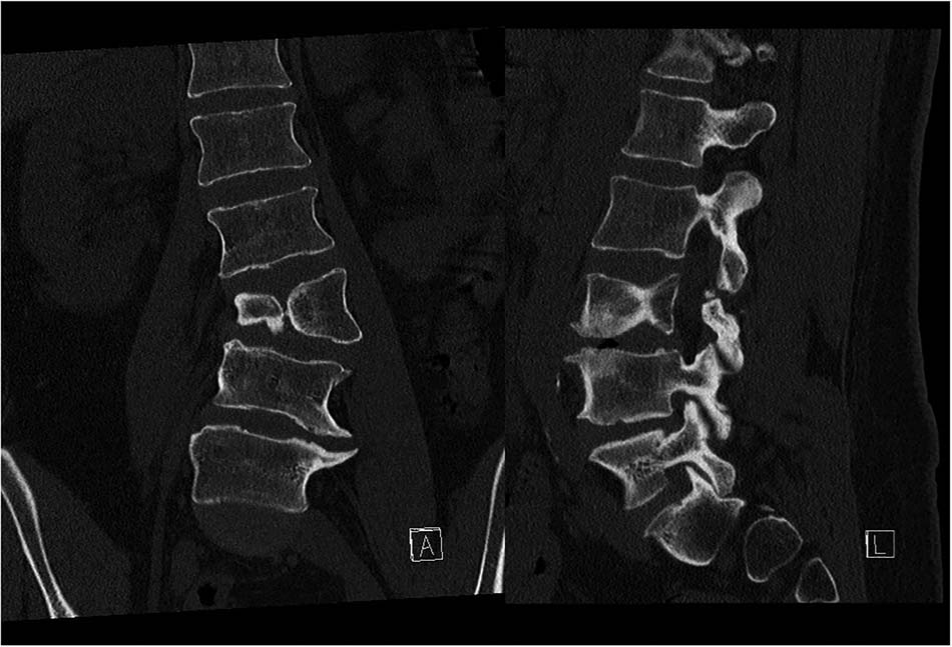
Preoperative CT demonstrating L3 hemivertebra; anteroposterior view (A) on the left side of the image, and lateral view (L) on the right side of the image.
